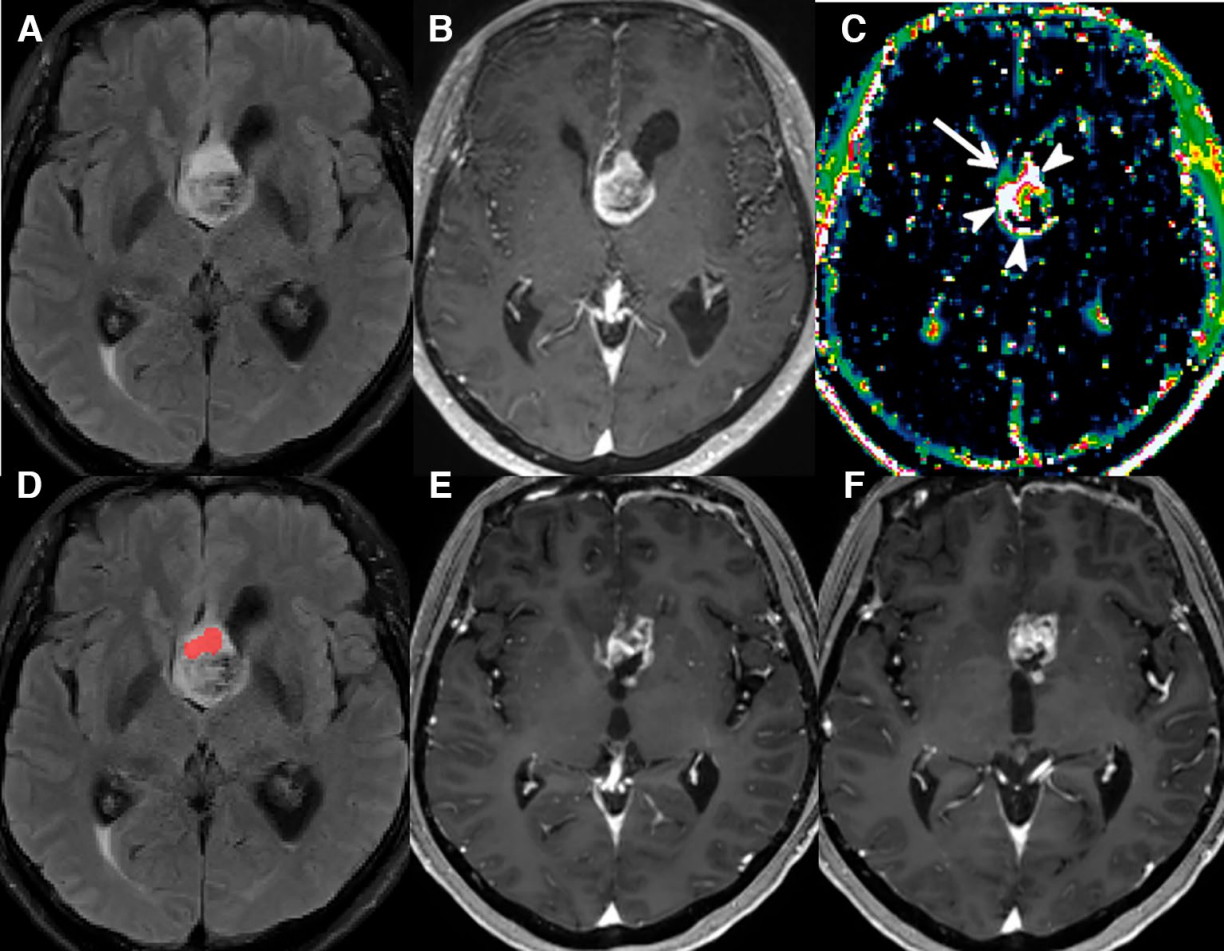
A 60-year-old woman with GBM in the training set. (A,B) Preoperative T2 FLAIR and CE T1W images show a heterogeneously enhancing mass at the septum pellucidum and corpus callosum with a small surrounding area of non-enhancing T2 hyperintensity. (C) The V_e_ map depicts a mild increase at the anterior aspect of the enhancing tumor (arrow) along with an overt increase at the enhancing portion of the tumor (arrowheads). (D) The combined MR model predicted local recurrence to occur at the non-enhancing T2 hyperintense lesion anterior to the enhancing tumor (red area). (E,F) At the 7-month follow-up, a measurable enhancing lesion appeared at the corpus callosum (genu). *GBM* glioblastoma, *FLAIR* fluid-attenuated inversion recovery, *CE T1W* contrast-enhanced T1-weighted.
